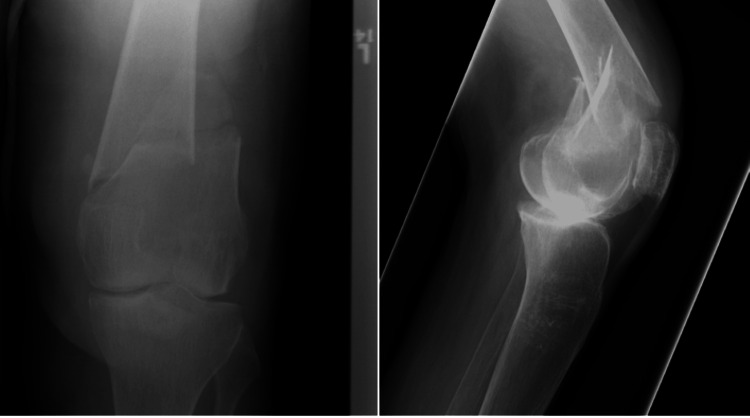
Case 4 preoperative X-rays: anteroposterior and lateral views
